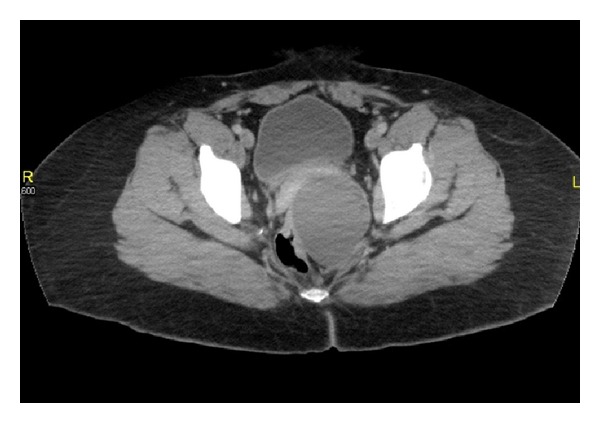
CT scan showing 7.5 × 6.4 × 6.6 cm abscess.
